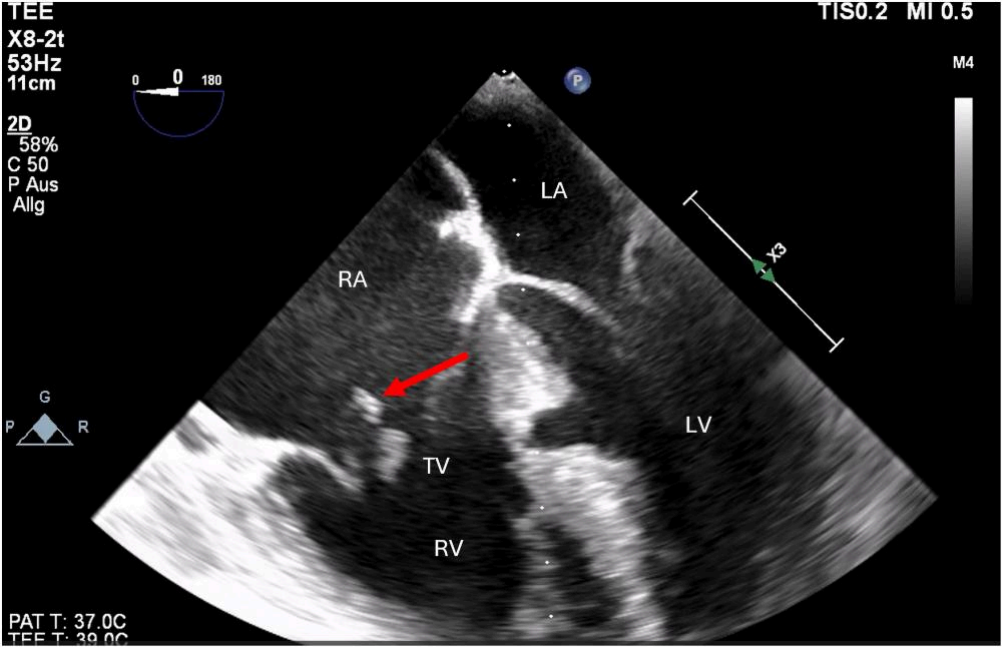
Transoesophageal echocardiography. Rupture of the tricuspid anterior papillary muscle (marked by red arrow); RA, right atrium; LA, left atrium; LV, left ventricle; RV, right ventricle; TV, tricuspid valve (©Medical University of Graz).
